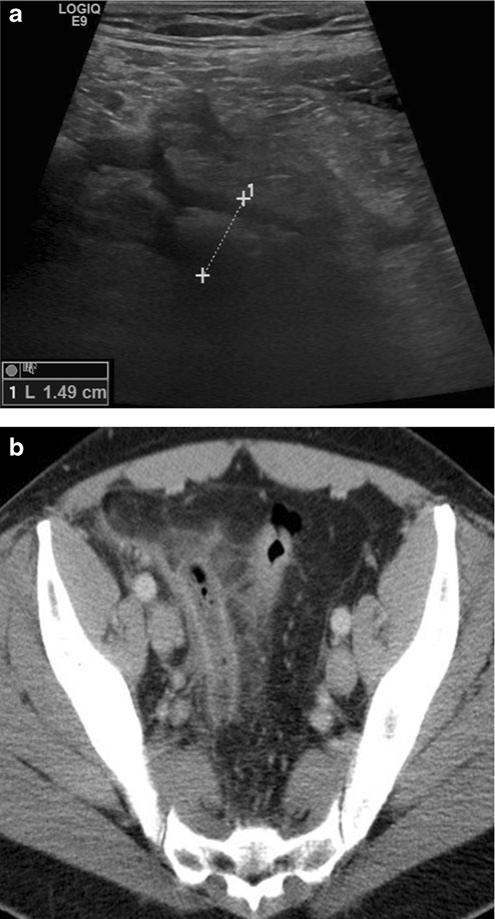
US and CT in acute appendicitis. 45-year-old male patient with pain in the right lower quadrant and increased inflammation parameters (white blood cell count and C-reactive protein elevation). a US real-time scan: local pain in combination with some fluid and thickened appendix, only seen in part (between *crosses*). b contrast-enhanced CT: thickened appendix, mesenteric infiltration around the appendix, inflammatory thickening of the sigmoid colon
